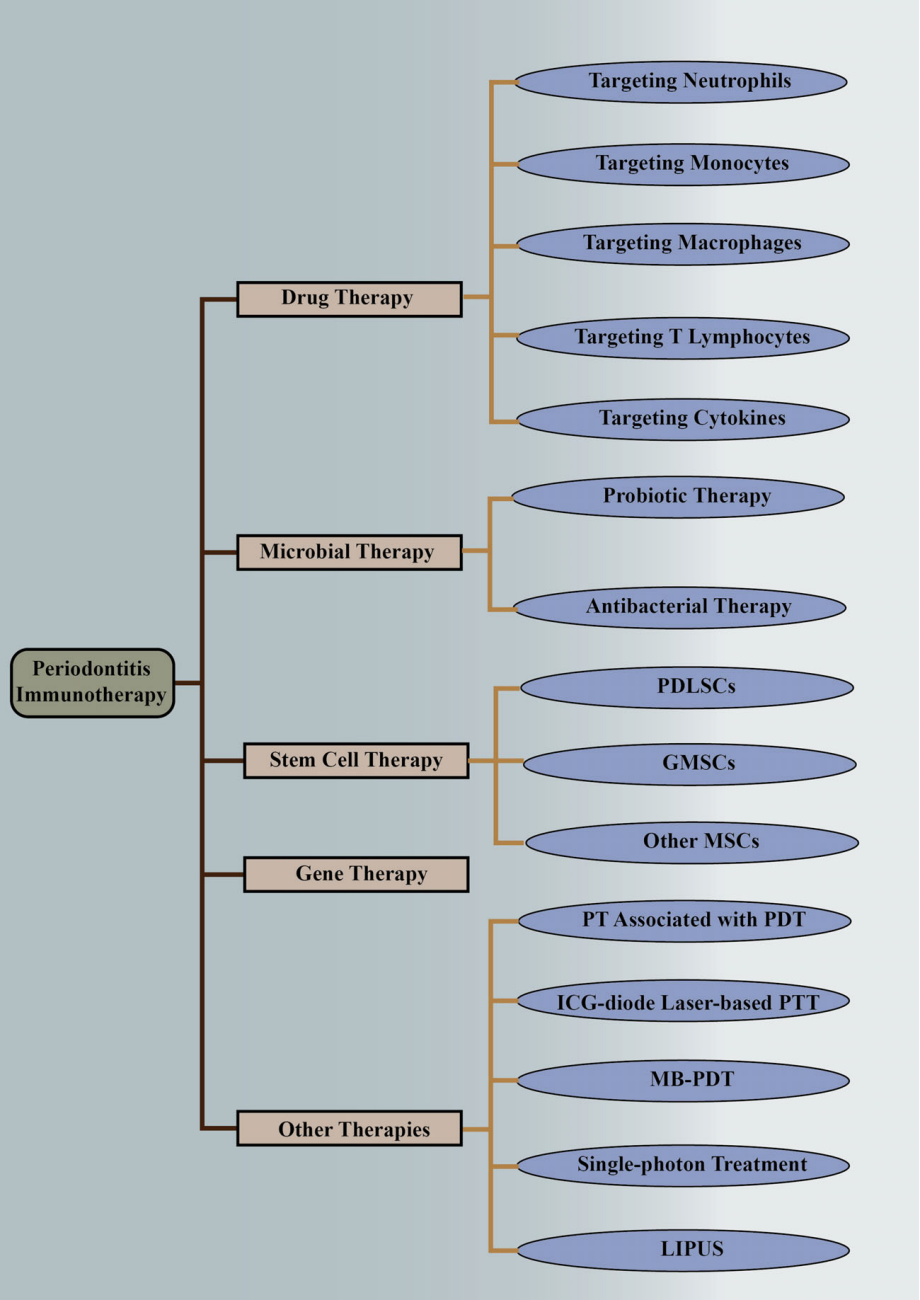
Immunotherapies of periodontitis. The above is a detailed classification of periodontitis immunotherapy, including drug therapy, microbial therapy, stem cell therapy, gene therapy, and other therapies. They are followed by more detailed classifications. PDLSCs, periodontal ligament stem cells; GMSCs, gingival mesenchymal stem cells; MSCs, mesenchymal stem cells; PT, periodontal therapy; PDT, photodynamic therapy; ICG, indocyanine green; PTT, photothermal therapy; MB-PDT, methylene blue-mediated photodynamic therapy; LIPUS, low-intensity pulsed ultrasound.
